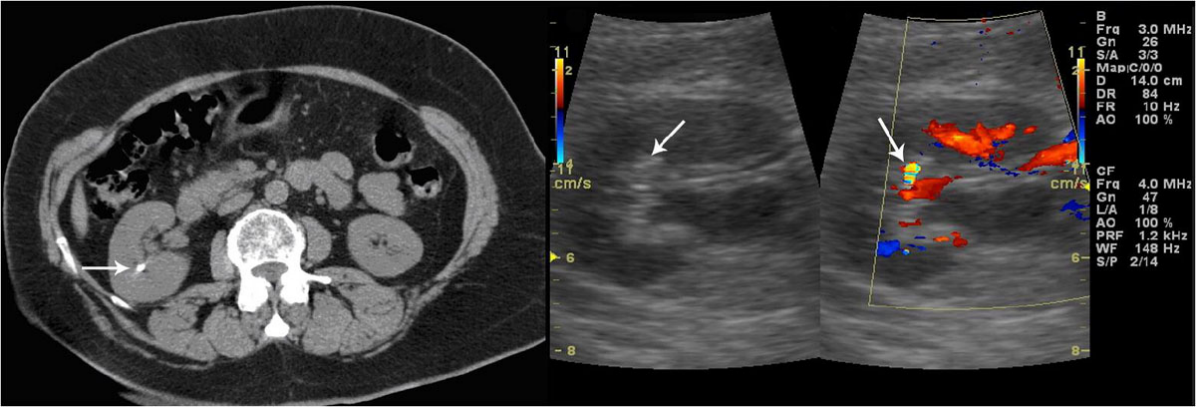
Unenhanced CT confirms the presence of renal stone. Grey-scale sonogram shows small hyperechoic spot without posterior acoustic shadowing. Color Doppler sonogram shows a twinkling sign.
